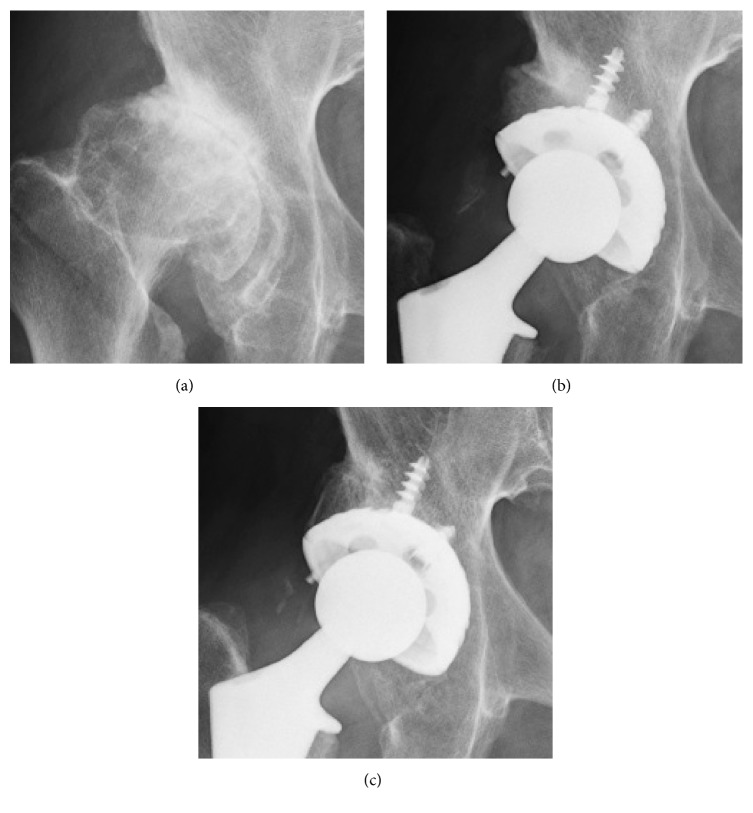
(a) Preoperative radiograph of a 54-year-old woman with Crowe type II dysplasia. (b) On a postoperative radiograph, the cup center-edge angle was −9.2° and the bone coverage index was 48.8%. A morselized autograft was used. (c) A radiograph taken 3 years after surgery showed fixation by bone ingrowth with no radiolucent lines. The allograft was perfectly incorporated into the host bone.
